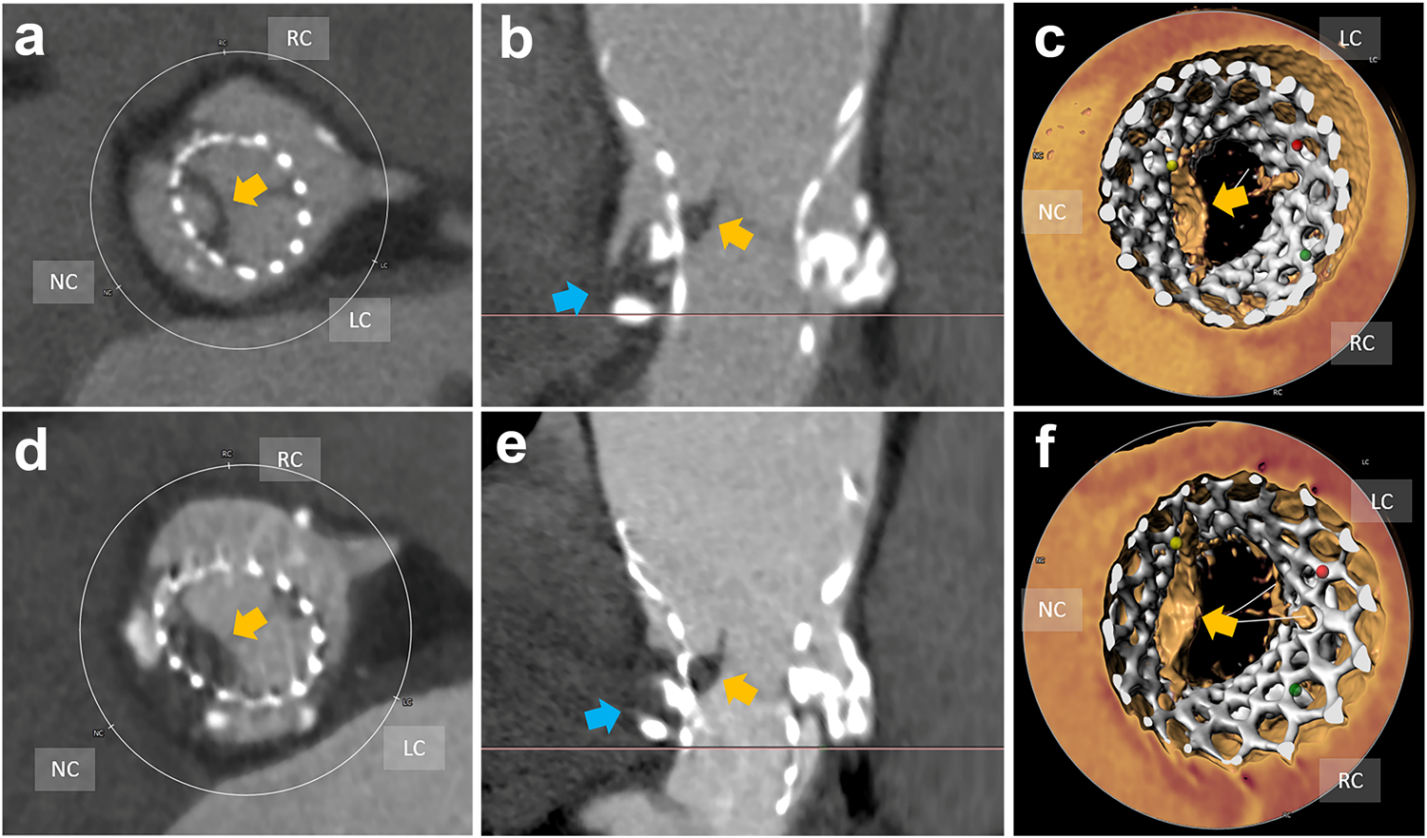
HALT score 2–3 and persistent SFD: a The non-coronary leaflet thickening (yellow arrow) on the short-axis view in 5-year follow-up cardiac CTA after TAVI, (b) The leaflet was hypoattenuated thickening confined to half of the leaflet (yellow arrow) on the long-axis view, and SFD was found in the non-coronary sinus (blue arrow), (c) thickening of the left leaflet (yellow arrow) on VRT reconstruction image. d, e The non-coronary leaflet thickening increased to about 3/4 of the leaflet (yellow arrow) in the 6-year CTA, SFD was also progressed (blue arrow). f Leaflet thickening is more obvious (yellow arrow) in the 6-year CTA than that in the 5-year CTA
